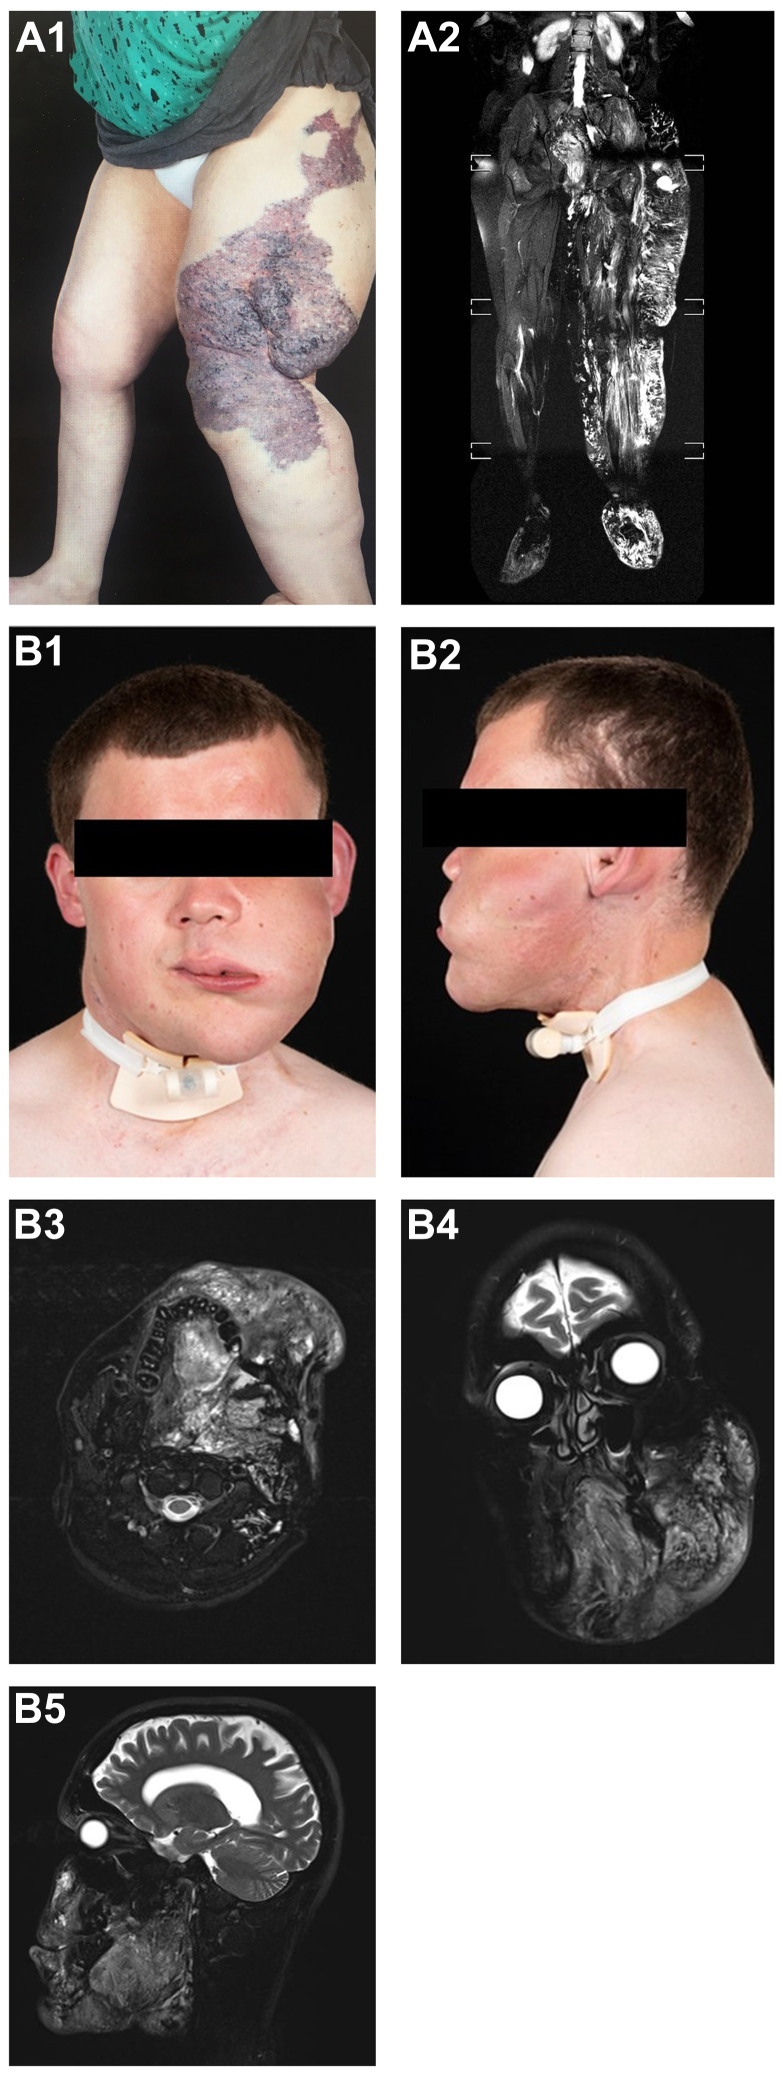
Medical photography and MRI of a 53-year-old woman lower limb Klippel-Trenaunay syndrome (KTS) (A1-A2) and of 23-year-old man a with facial malformation (B1-B5).
